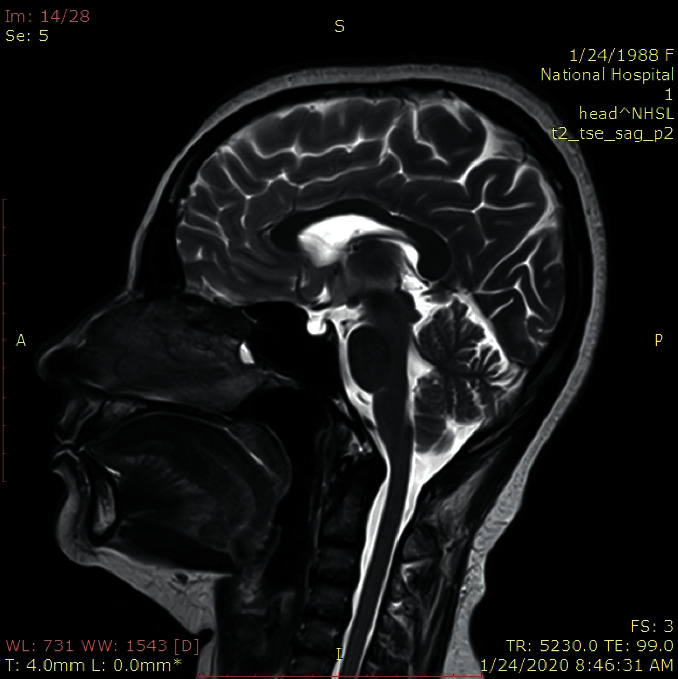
MRI-pituitary of the patient showing empty sella. T2 sagittal section of MRI-sellar region shows normal-sized pituitary fossa filled with CSF. The pituitary gland is not seen in the sella.
